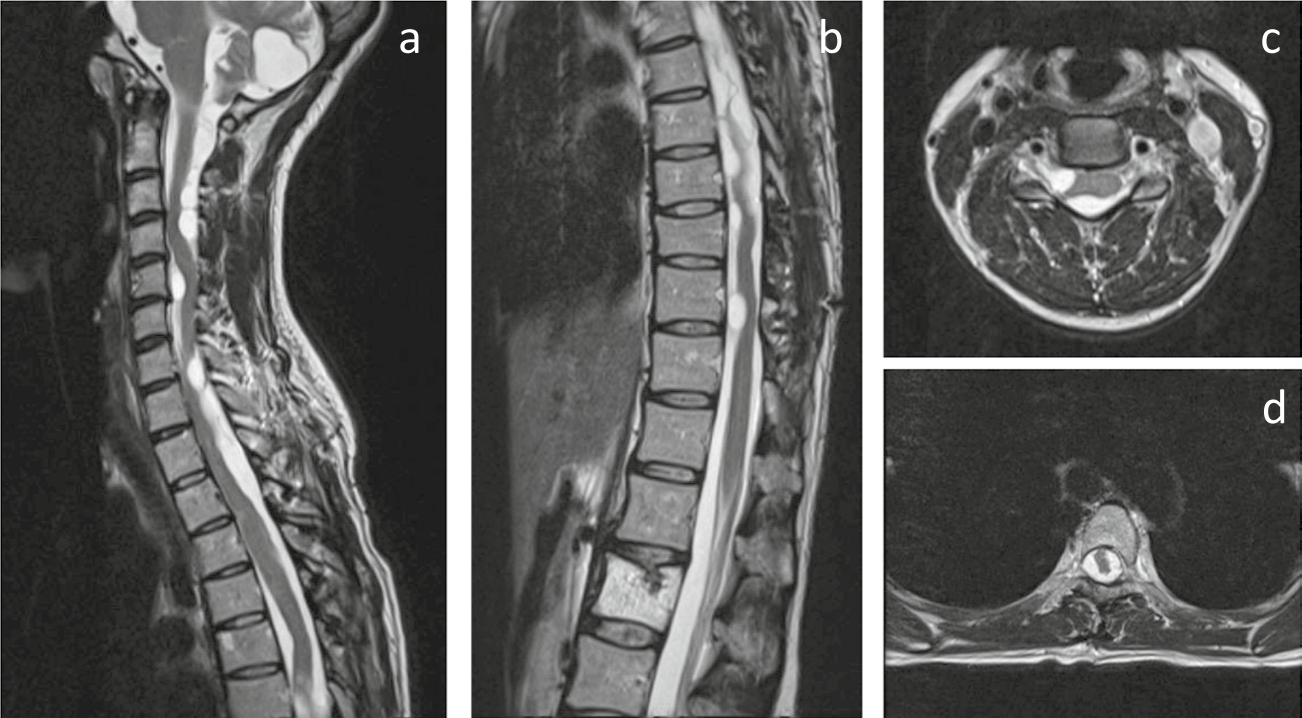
Sagittal MRI sections of the a cervical and upper thoracic and b the lower thoracic spine. Axial MRI sections at c C3 and d Th4, 3 years after presentation
